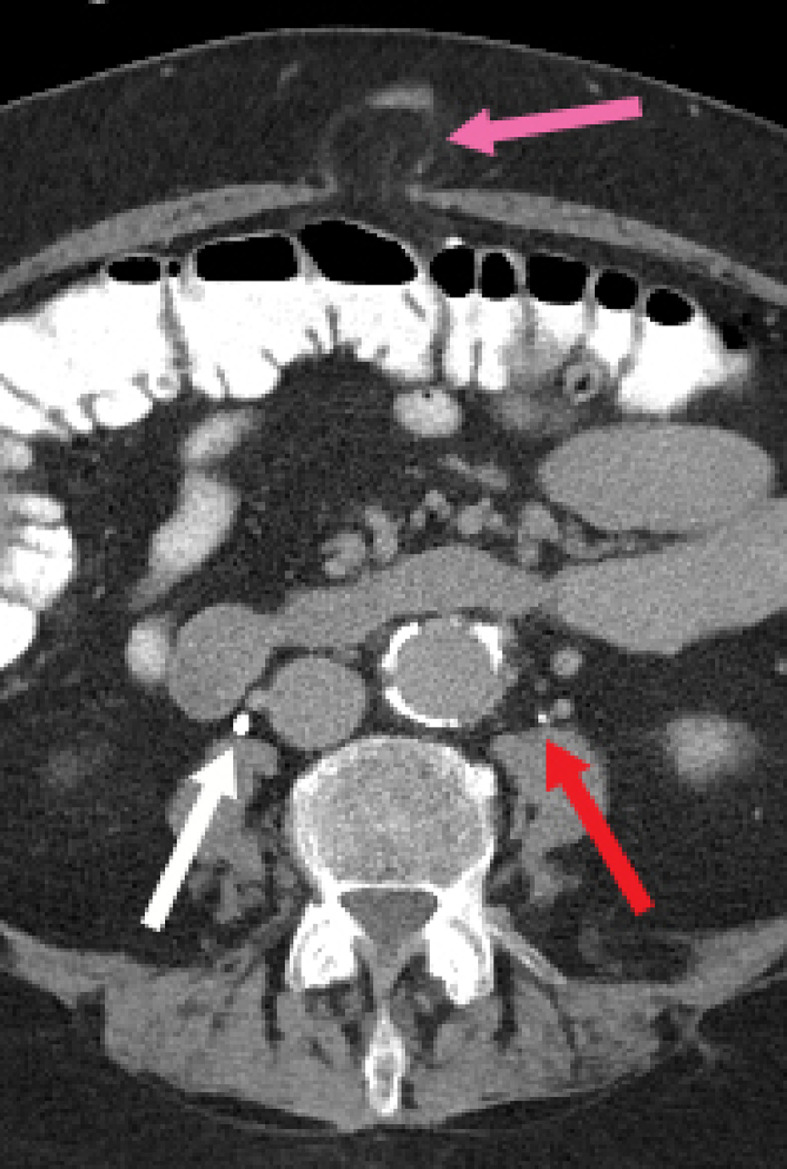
Axial CT image demonstrates a fat-containing umbilical hernia (pink arrow). The opacified ureters overlie the psoas muscles just inferior to the level of the kidneys (white and red arrows) (color version of figure is available online).
